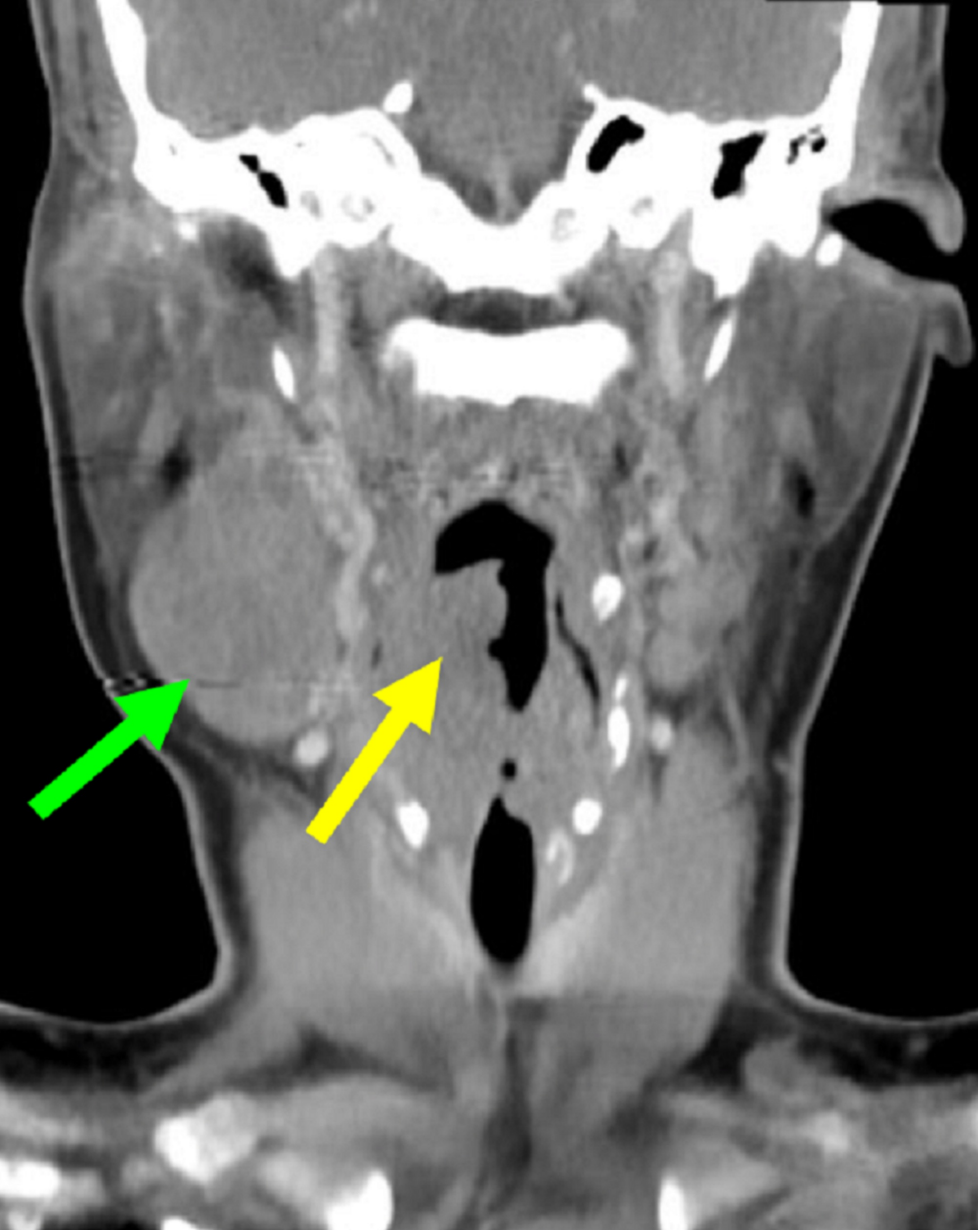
Coronal contrast-enhanced computed tomography (CT) image of the neck. Green arrow labels the right enlarged cervical lymph node. Yellow arrow indicates the primary hypopharyngeal mass.
